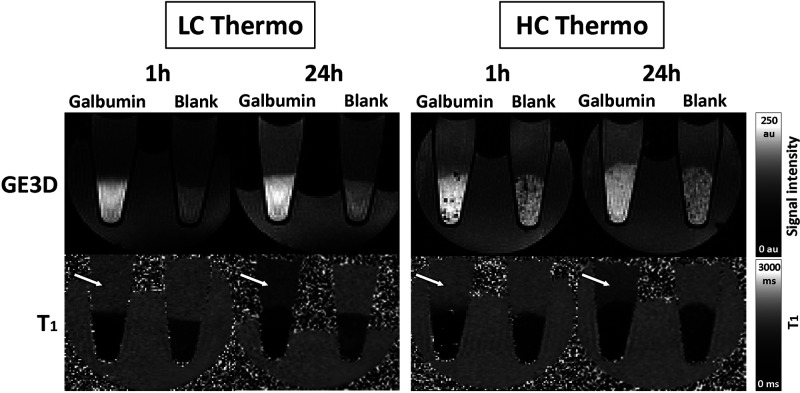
MRI of protein release from *in vitro* hydrogel
